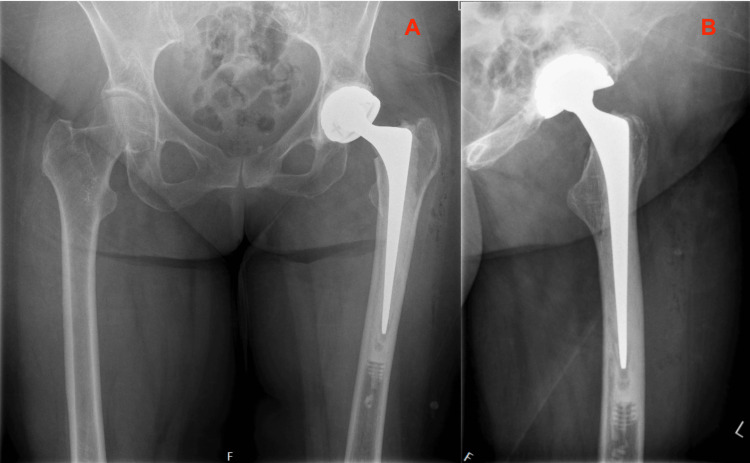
Primary Total Hip Arthroplasty Routine direct postoperative radiographs of the primary THA with CPT femoral stem, performed for a femoral neck fracture. 1A: Anterior Posterior view of the Pelvis; 1B: Lateral view of the Left Hip THA: Total Hip Arthroplasty; CPT: Collarless, Polished, Double-Taper
